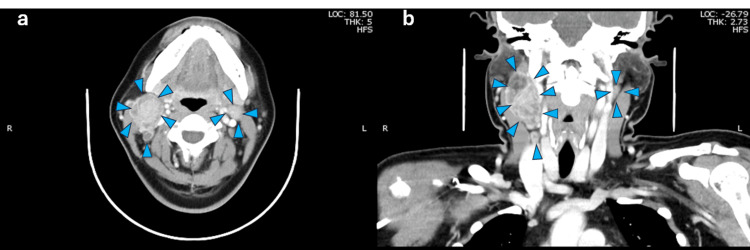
Contrast-enhanced computed tomography in case Figure 3a: Axial view, Figure 3b: Coronal view Bilateral lymph node metastasis are surrounded by blue arrowheads
